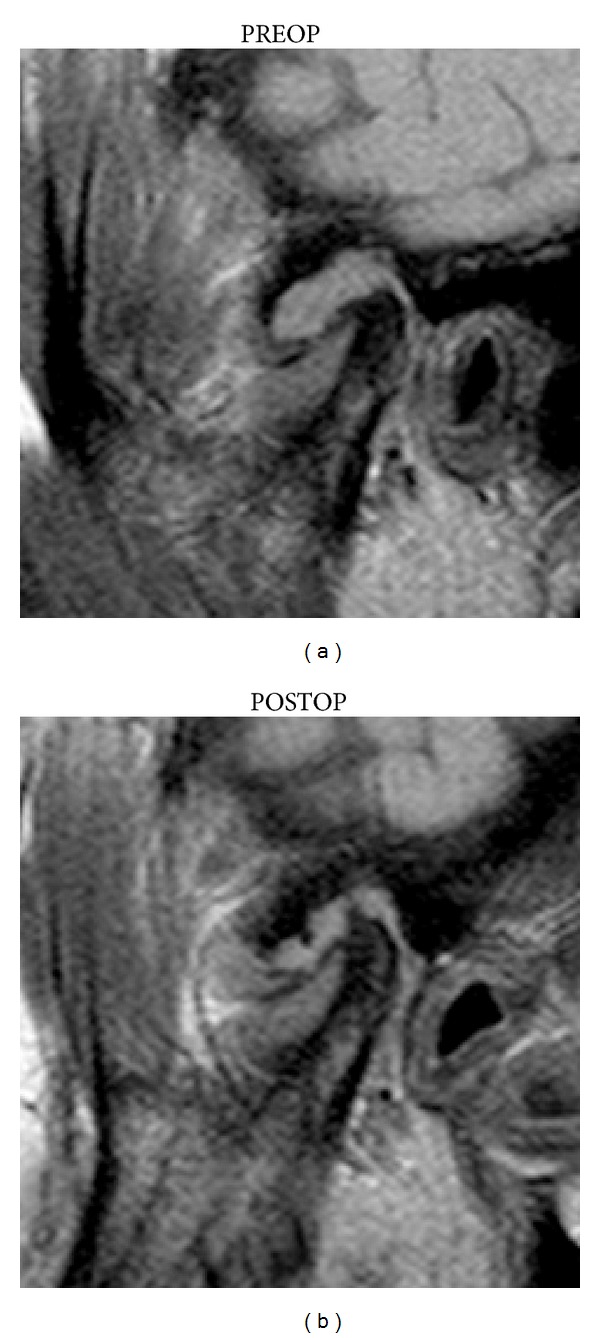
MRI: JE was identified as a high signal intensity area in the region of the joint space; note the mild reduction of inflammatory signs.
